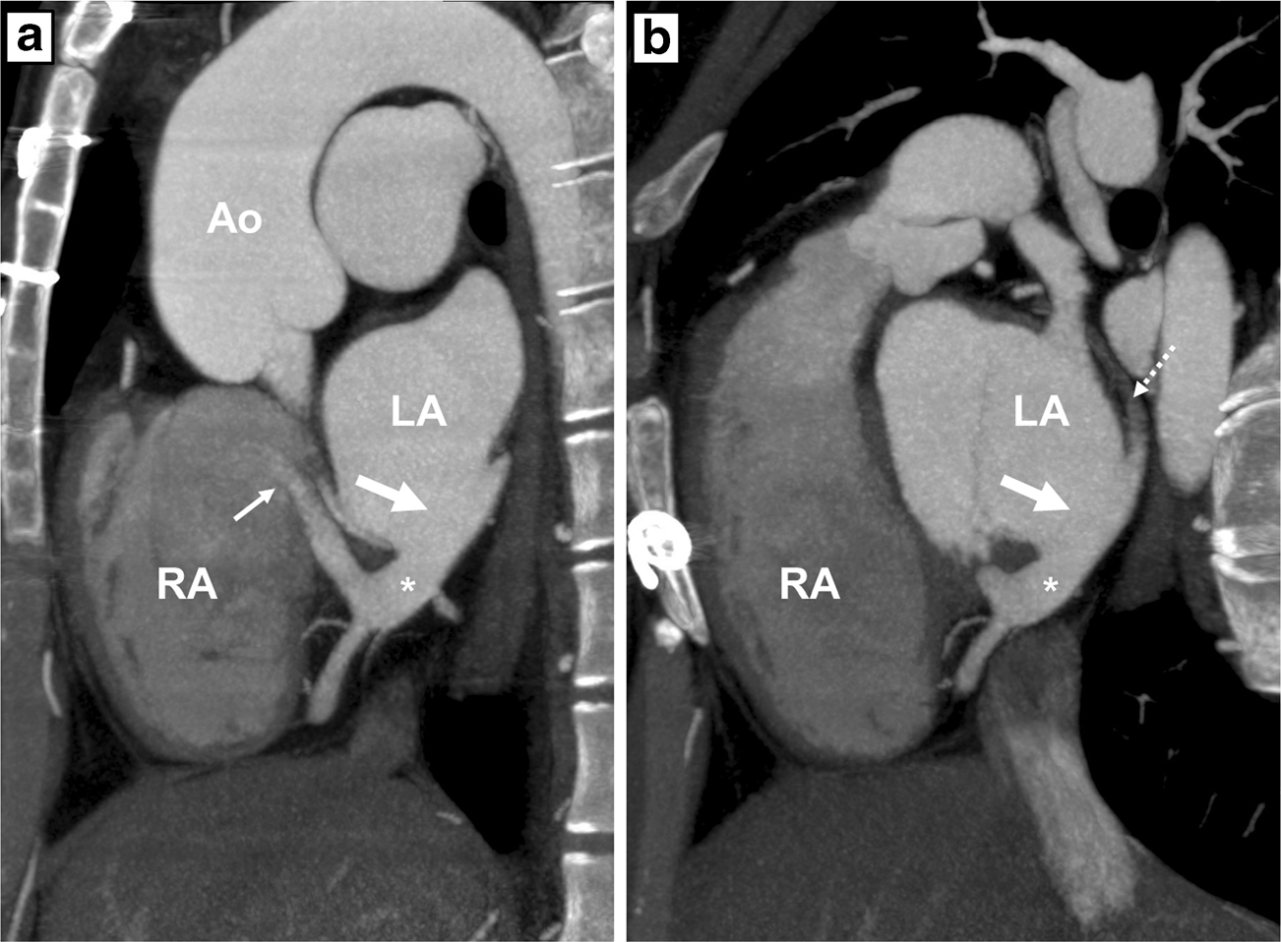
Computed tomography angiography (CTA) images after intravenous contrast administration in patient A. a: Sagittal oblique multiplanar reconstruction of the unroofed coronary sinus (*), the coronary sinus defect (*thick arrow*) and the entry of the coronary sinus into the right atrium (*thin arrow*). b: Note that the great cardiac vein is not opacified by intravenous contrast (*dotted arrow*) and that the coronary sinus shows opacification comparable to the left atrium. *RA* right atrium, *LA* left atrium, *Ao* aorta
